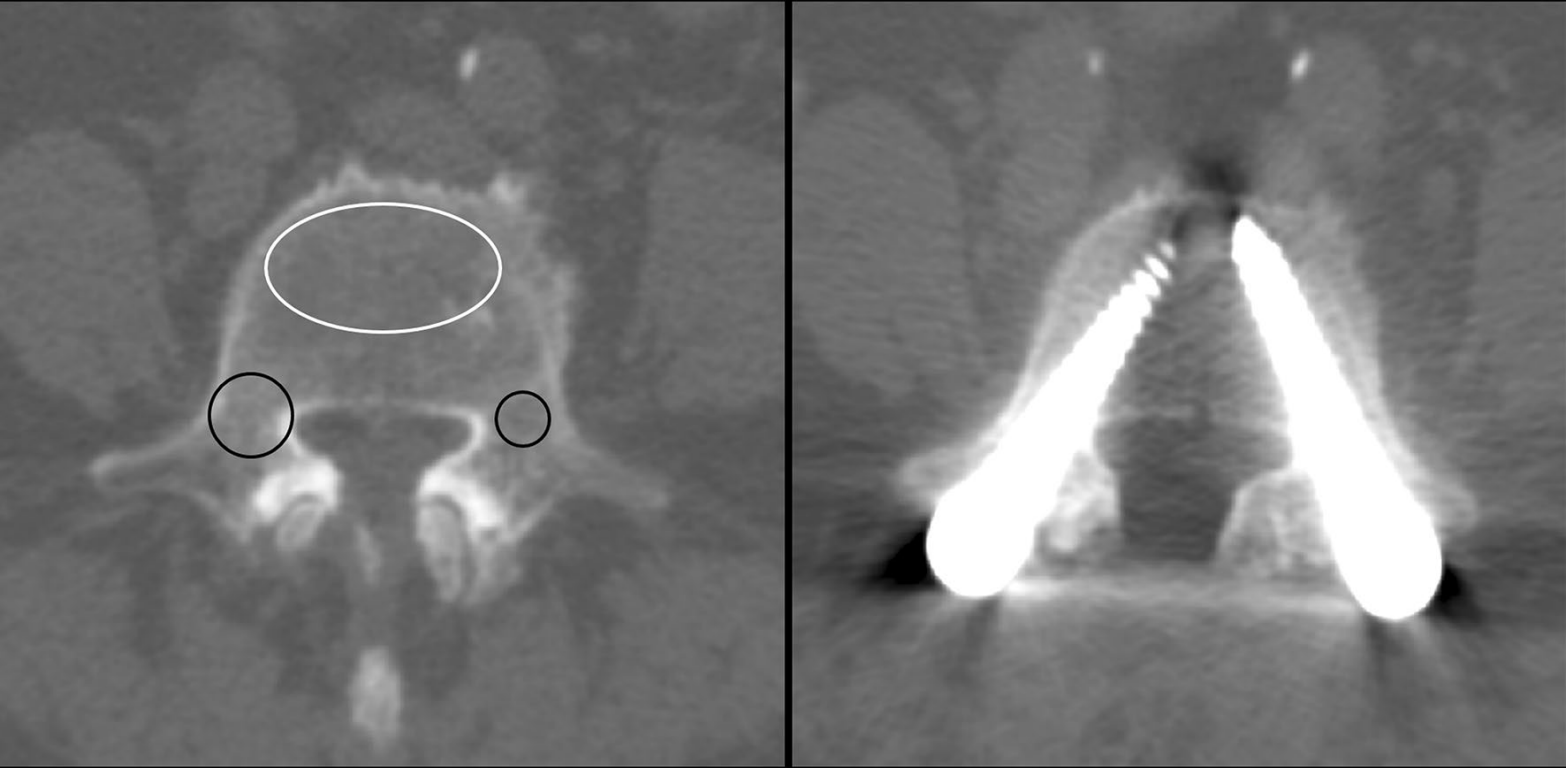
A 69 year-old female underwent Dynesys dynamic stabilization (DDS) system at L3–4–5. Left*:* the measurement of the CT Hounsfield Unit (HU) values of the L5 vertebral body (VB, white circle) and pedicles, including (large black circle at the right pedicle) and excluding the cortical bone (small black circle at the left pedicle) in this patient. The HU value of the VB was 124. The HU values of the right pedicle including and excluding the cortical bone were 206 and 156, respectively. The left HU values of the left pedicle including and excluding the cortical bone were 250 and 180, respectively. Right: although all HU values measured on pre-operative CT in this patient were lower than the mean HU values in our cohort, post-operative 24 months CT demonstrated no screw loosening at L5.
